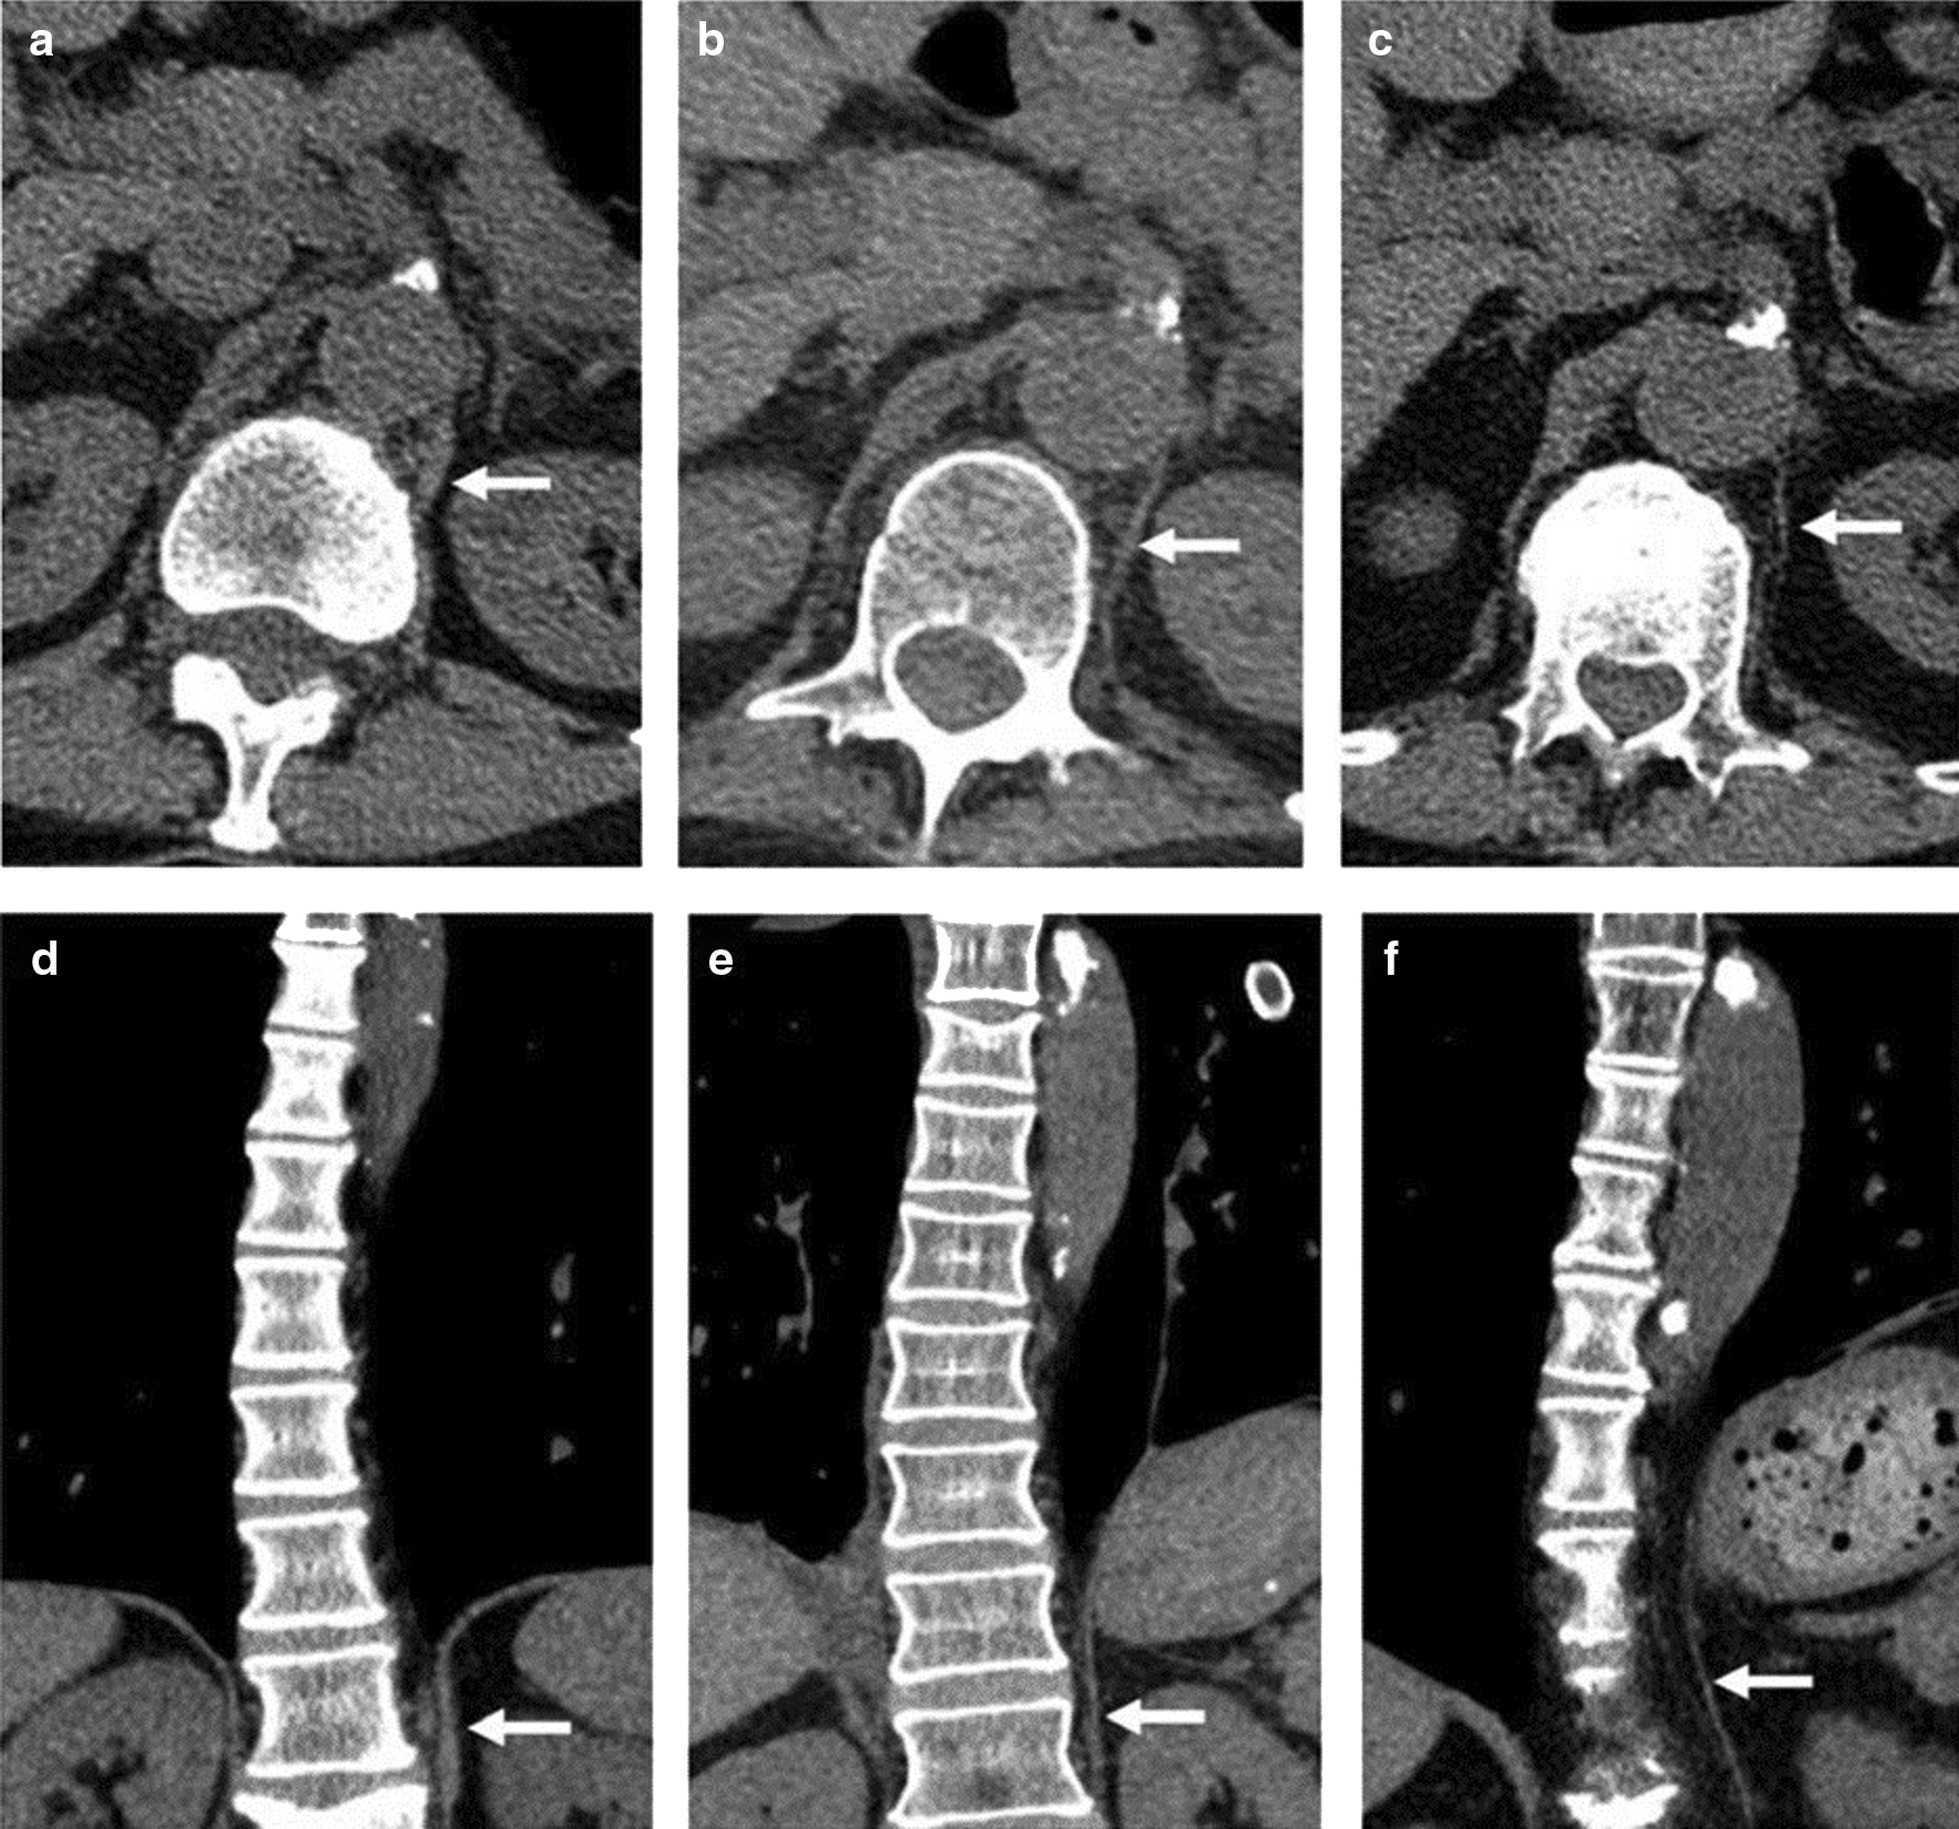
Progressive hemidiaphragm thinning after double lung transplantation for COPD. Axial (a–c) and coronal (d–f) CT images pre-transplantation (*left panels)*, 1 month after transplantation *(middle panels)* and 11 months after lung transplantation (*right panels)*. The pre-transplantation images demonstrate equal crural diaphragm thickness; the left hemidiaphragm (white arrow) becomes progressively thinner and moves cranially after transplantation. These findings are indicative of post-operative left hemidiaphragm paralysis. Reproduced with permission from Wolters Kluwer Health: Sukkasem et al. Journal of Thoracic Imaging 2017;32(6): 383–390
